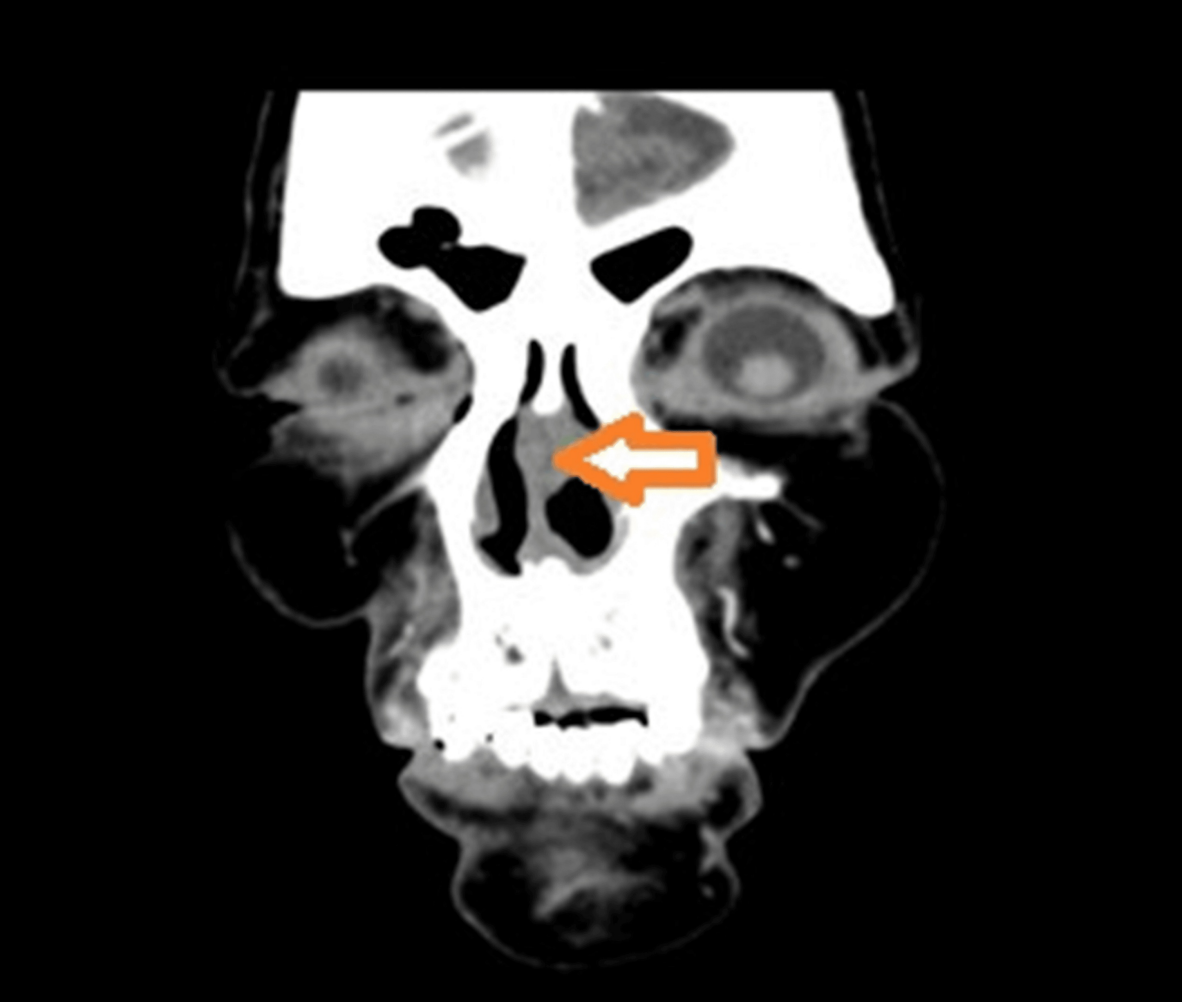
CT PNS showing soft tissue opacification of left side nasal septum (orange arrow) CT: computed tomography; PNS: paranasal sinuses
